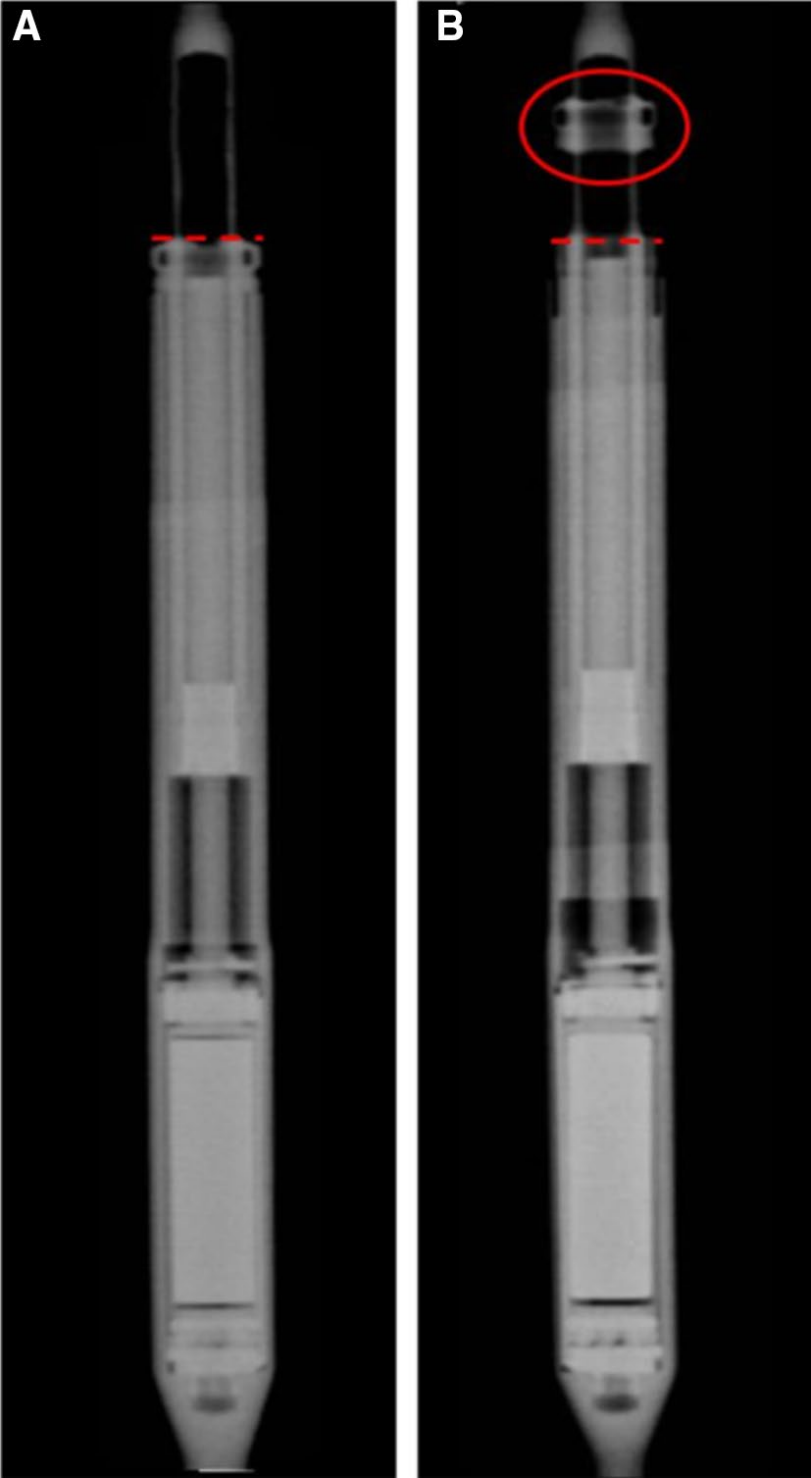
Example X-ray images of (a) a rod with a well-fixed end cap and (b) a rod with a separated end cap [3]
